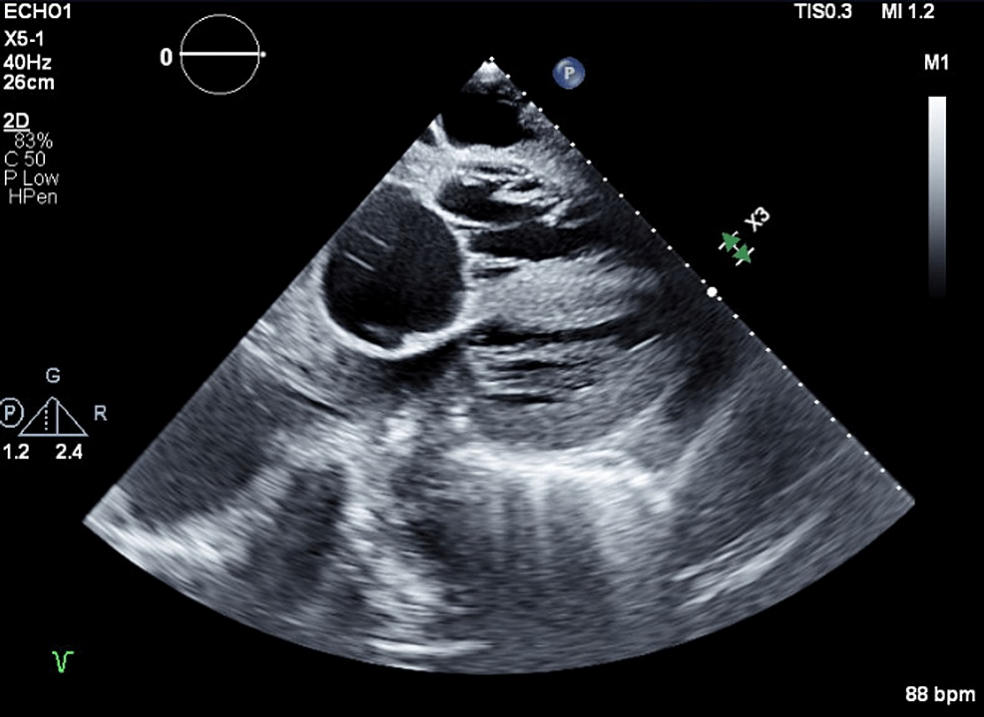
Subcostal View - Transthoracic Echocardiogram
